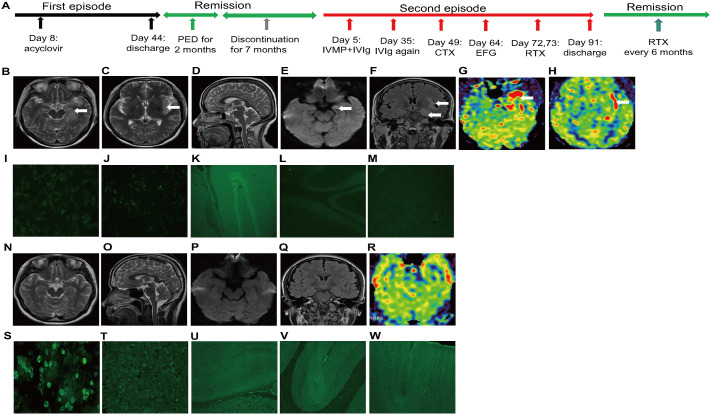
Clinical timeline, neuroimaging features and antibody profiles in a patient with relapsing anti-NMDAR encephalitis. (A)​ Disease course. The first episode presented with left temporo-insular lesions and hyperperfusion [(B-H), arrow] alongside low-level antibodies [serum/CSF: 1:10/1:3.2; (I, J)] and a positive tissue-based assay (K-M). The second, severe relapse demonstrates a key clinical-radiological dissociation: MRI was normal (N-R) despite catastrophic symptoms and significantly elevated antibody titers [1:100/1:32; (S, T), with positive TBA (U-W)].​ The clinical response to EFG-RTX occurred in this context of seropositive, MRI-negative disease activity. CSF, cerebrospinal fluid; CTX, cyclophosphamide; EFG, efgartigimod; IVIg, intravenous immunoglobulin; IVMP, intravenous methylprednisolone; NMDAR, N-methyl-D-aspartate-receptor; PED, prednisone; RTX, rituximab.
